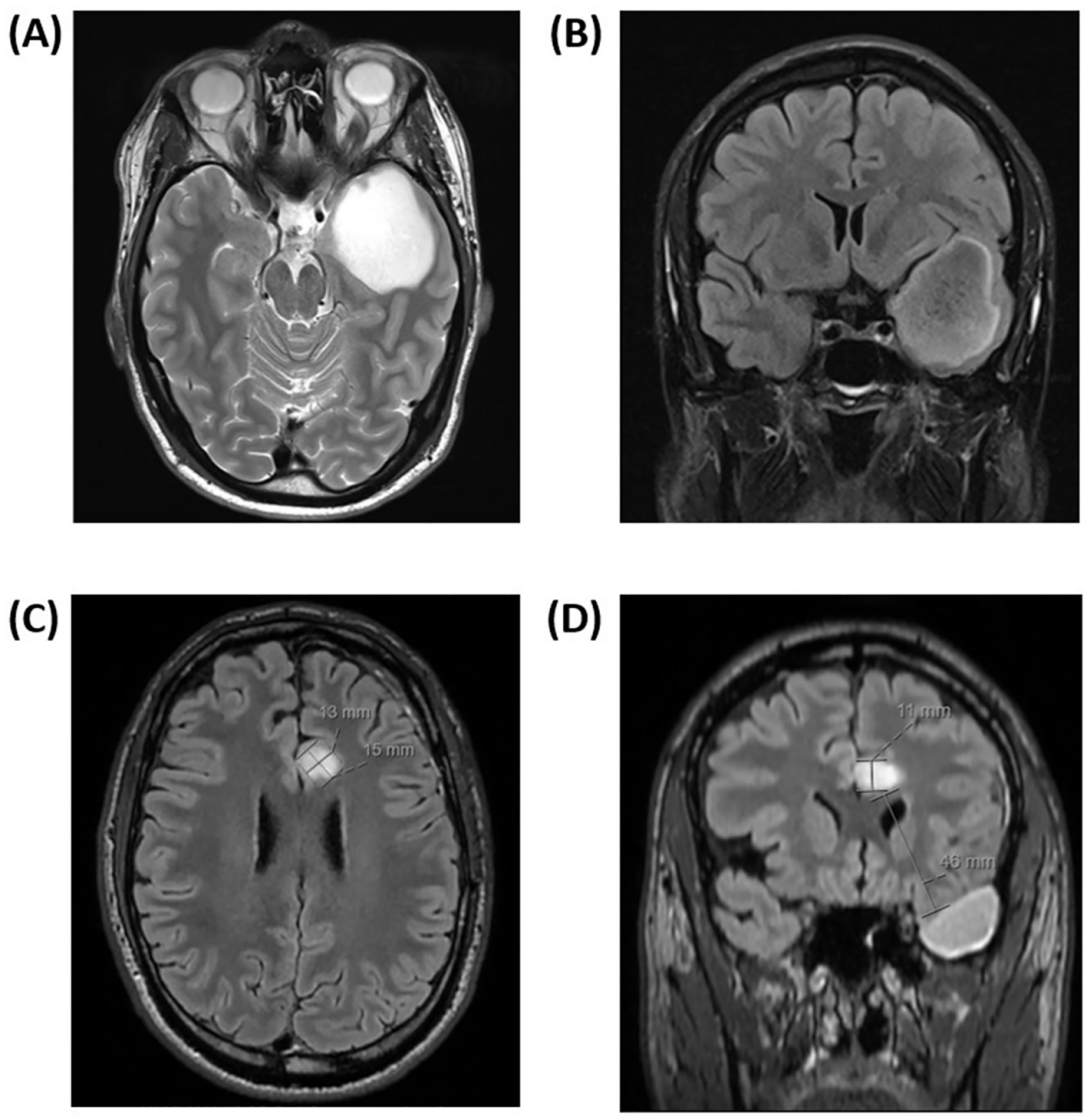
(A, B) Axial magnetic resonance contrast-enhanced T2/FLAIR image sequence prior to the first tumor resection showed a nonenhancing cystic lesion in the left temporal lobe, suspicious for diffuse low-grade glioma. The tumor measured 5 x 4 x 5 centimeters in its largest dimension. (C, D) Axial magnetic resonance contrast-enhanced T2/FLAIR image sequence three months after completion of proton beam therapy. A new 15 x 13 x 11 millimeters nonenhancing lesion, highly suspicious of distant neoplastic recurrence in the left subcortical, parasagittal left limbic area was found. The appearance of the lesion was relatively well-defined and expansive, and it was located 46 millimeters from the resection cavity.
